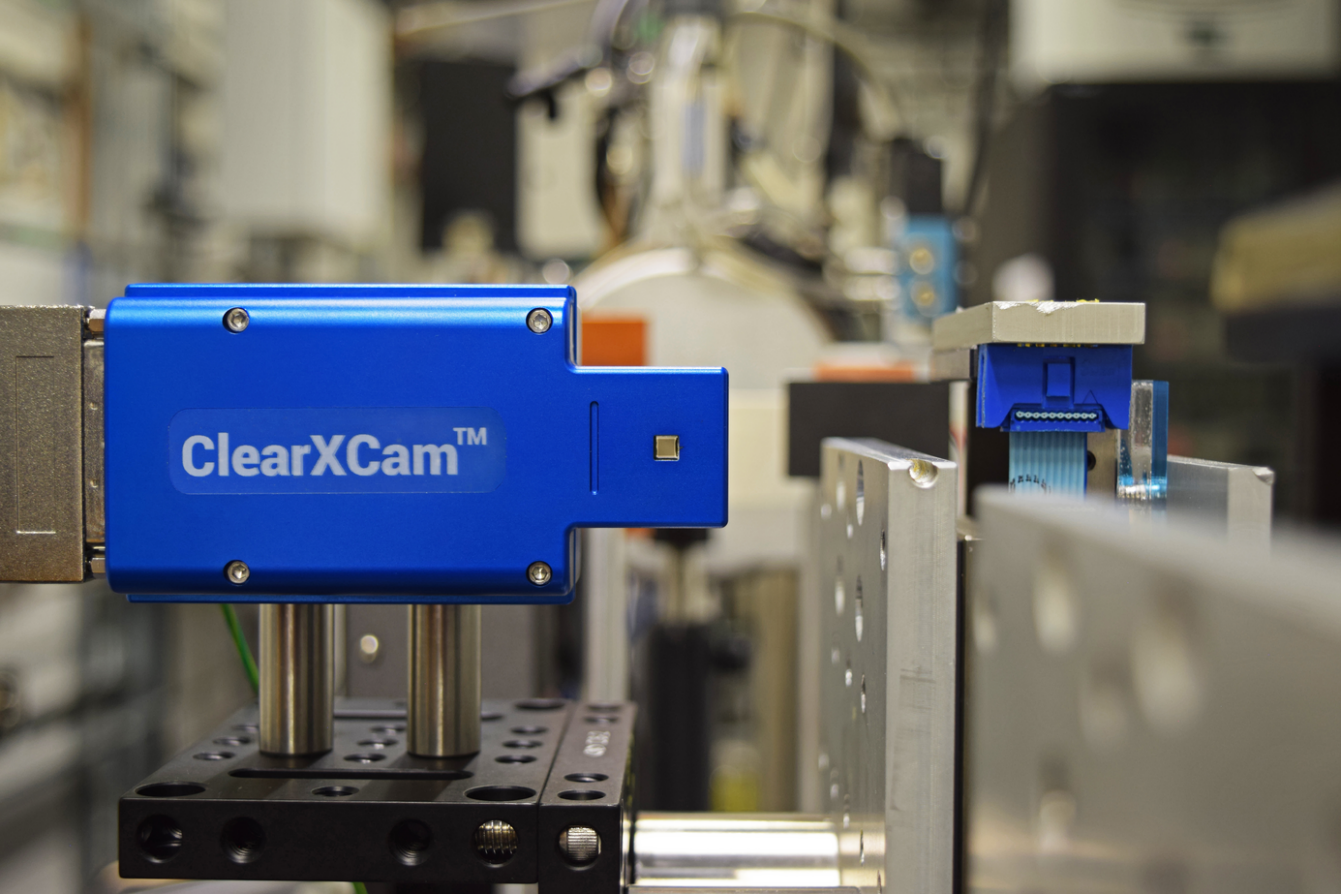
Photograph of the setup at the NSLS-II 17-BM beam­line. The ClearXCam sensor is positioned in the X-ray beam path and is mounted on a motorized stage for alignment and stability. This setup enables in-beam monitoring.
